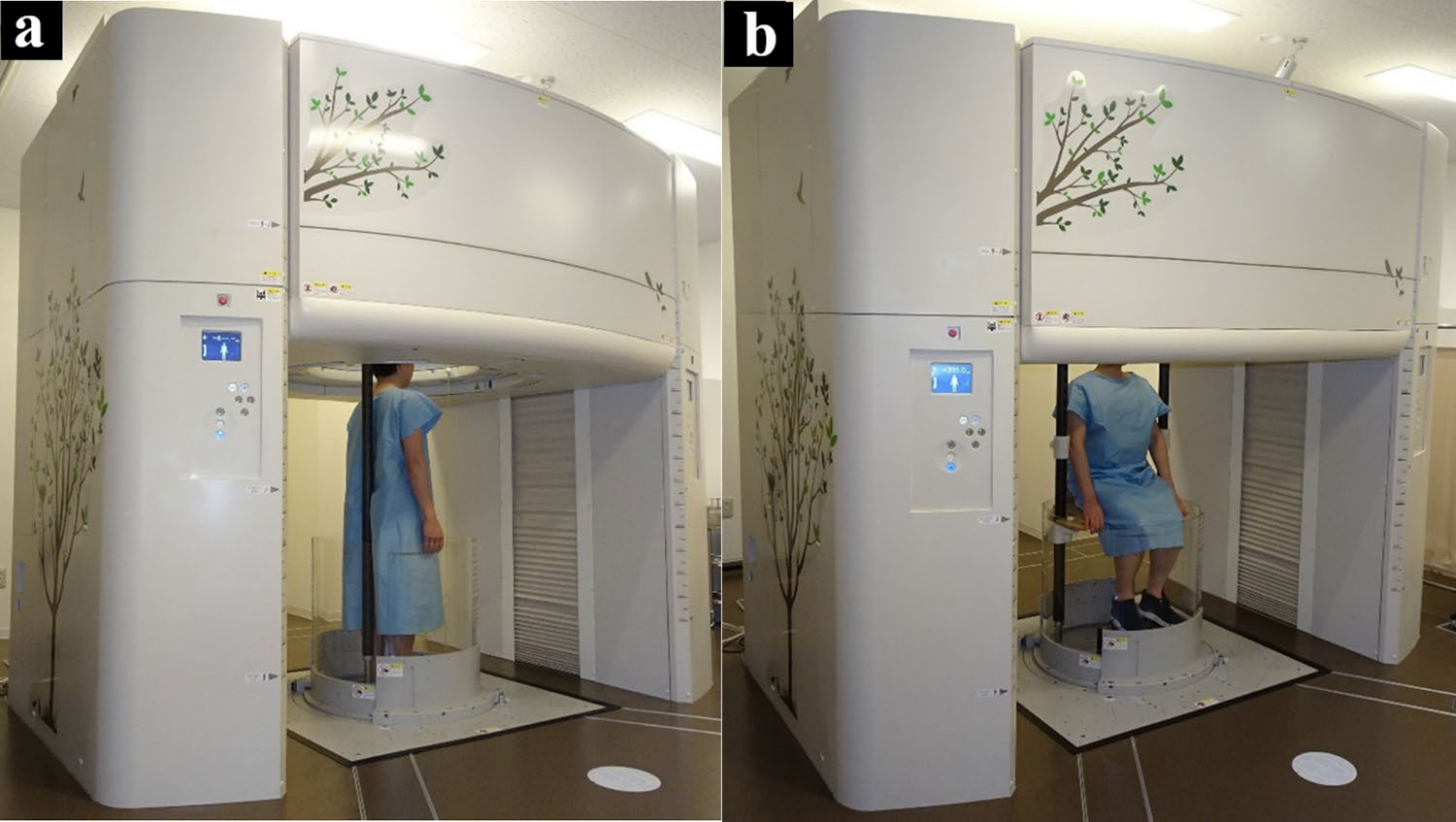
Upright CT examinations performed in the standing (a) and sitting (b) positions. The upright CT system features up-and-down movement of a transverse 320-detector-row gantry. For enhanced safety during upright scanning, an acrylic enclosure surrounds the subject to prevent falls, equipped with both a pinch prevention and contact interlock mechanism. To provide stability for standing subjects (a), a 2.3-m-long carbon back-support pole is installed, extending from the floor to the top of the system. This pole’s position is adjustable according to the subject’s body dimensions or specific scan requirements. In addition, for elderly or frail patients, a Velcro band can be attached to the pole and gently secured around the patient for support. As this subject is a healthy volunteer, the Velcro band is not applied
